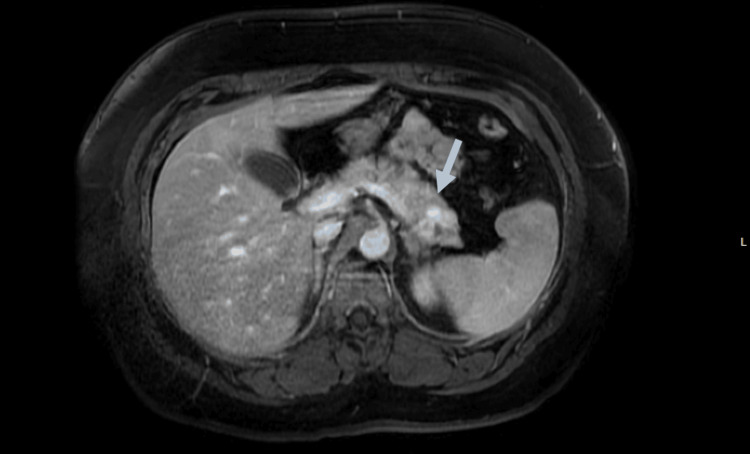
Trilobed pancreatic mass indicated by the white arrow on the abdominal magnetic resonance imaging (MRI).
